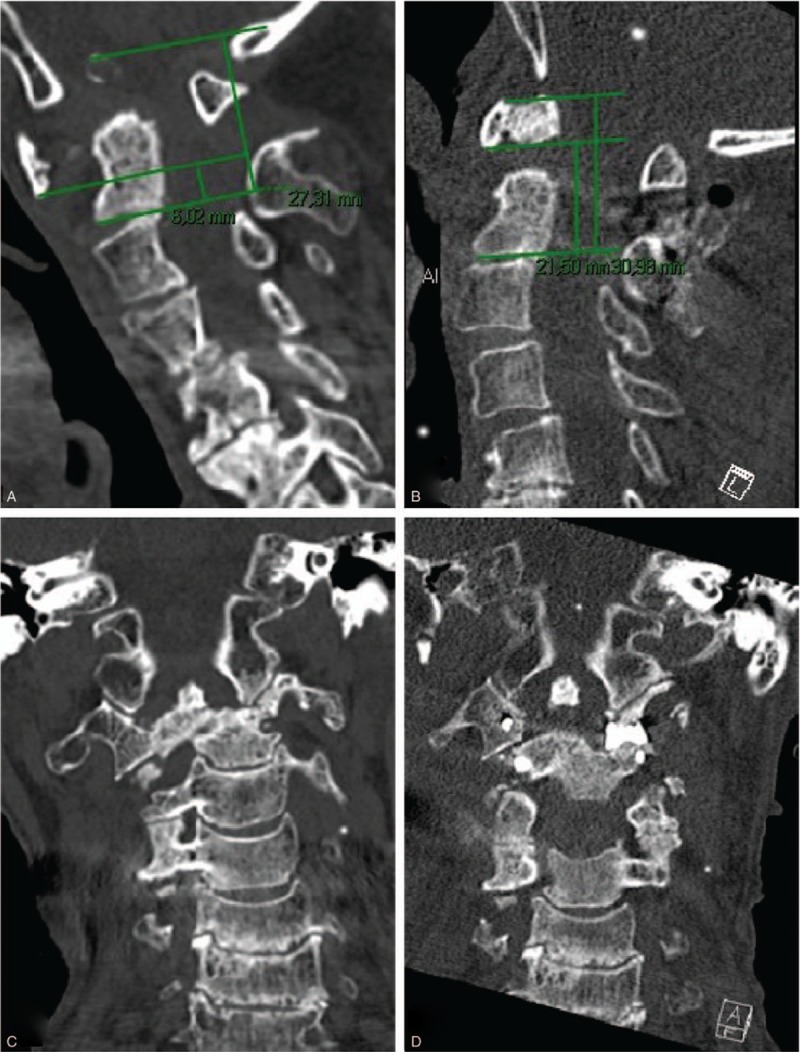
Images from case 1. (A) Preoperative sagittal CT view. The lines required to measure VAAI are shown. VAAI = 0.22. (B) Preoperative coronal CT view. The C1–C2 facet joint on the left side is severely damaged, with the axis shifting to the left. The axis is shifted posteriorly with basilar invagination. (C) Postoperative sagittal CT view. The lines required to measure VAAI are shown. VAAI = 0.69. (D) Postoperative coronal CT view. The C1–C2 facet spacer is inserted on the left side, improving coronal alignment. CT = computed tomography, VAAI = vertical atlantoaxial index.
